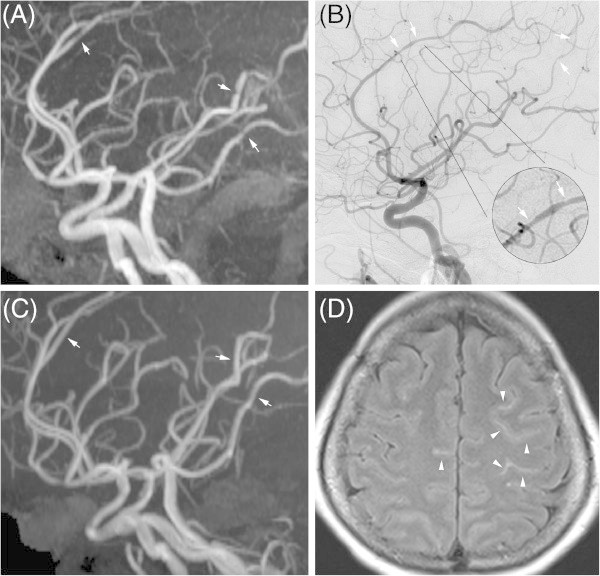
Imaging findings of reversible cerebral vasoconstriction syndrome. Multifocal vasoconstriction demonstrated by magnetic resonance angiography (MRA) (A) and catheter angiography (B), involving the anterior, middle, and posterior cerebral arteries. Follow-up MRA (C) revealed significant interval resolution of the previous lesions. Axial fluid attenuated inversion recovery (FLAIR) imaging revealed linear hyperintensity lesions in the sulci of the bilateral frontal lobes (D).
